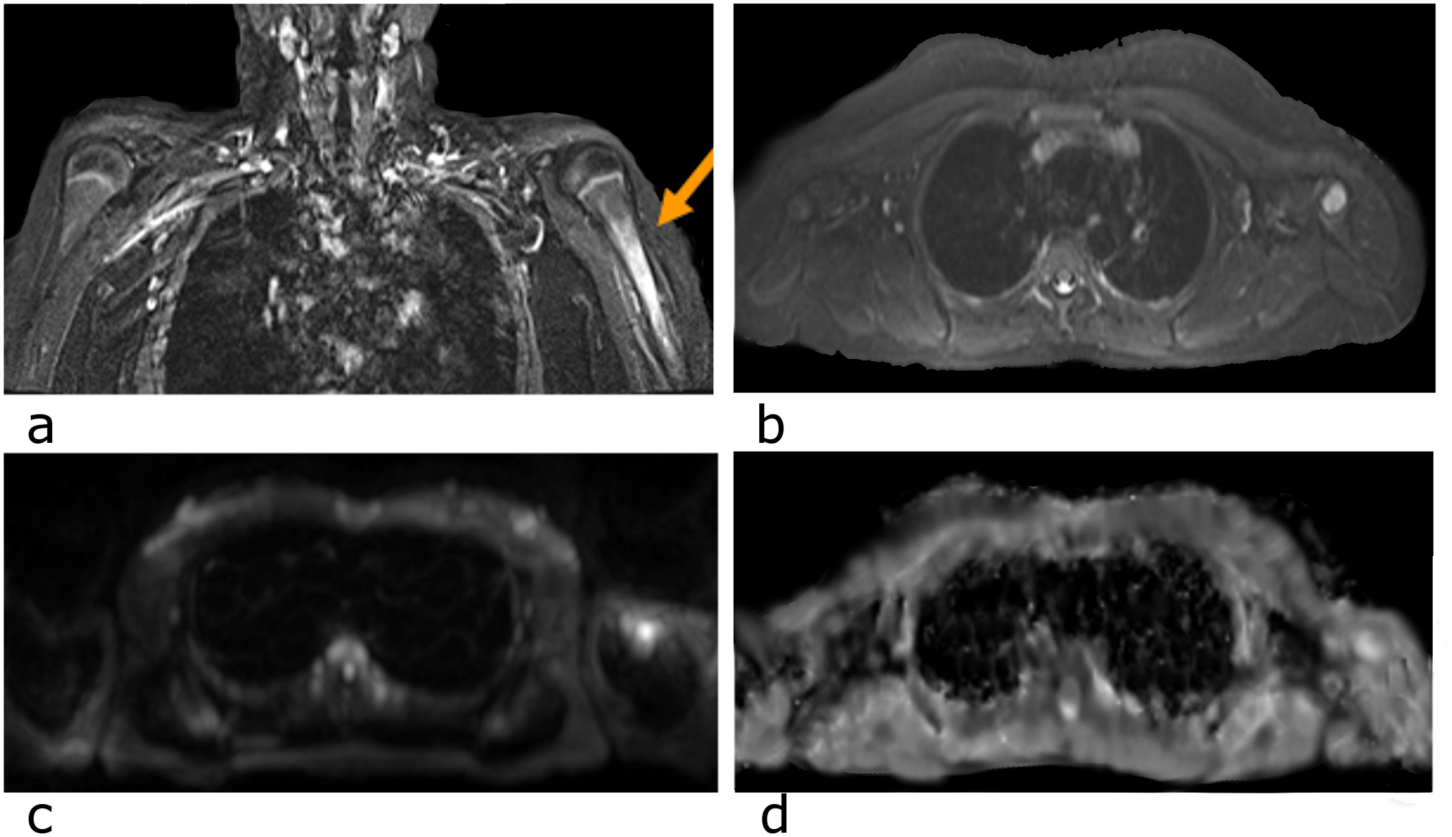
MR images of 12-year old girl with known CRMO. a) STIR-weighted images show diaphyseal edematous bone marrow involvement, periostal reaction of the left humerus b) axial series showing large and homogenous bony involvement c) signal alteration in DWI b = 800 images d) ADC increase at the corresponding site.
